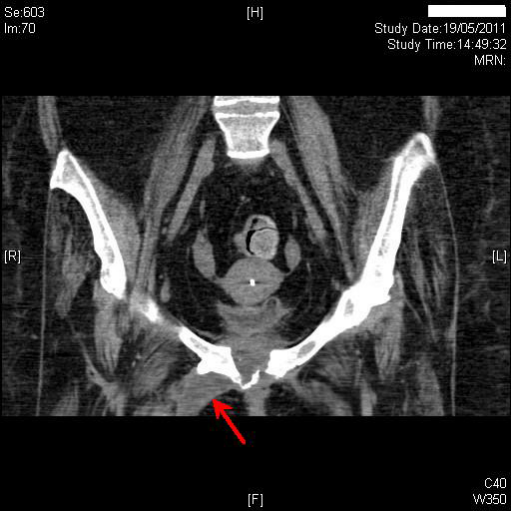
Coronal reformatted image of Computed tomography, performed eleven months after first CT, revealed collection of fluid surrounding right adductor muscle (red arrow).
